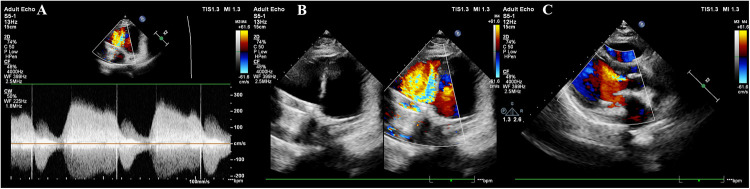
Cardiac ultrasound findings at 32 weeks of gestation. (A) Mechanical mitral valve with anterograde peak velocity (Vmax) of 2.4 m/s and mean pressure gradient (PGmean) of 6 mmHg; Tissue Doppler imaging shows mitral annular diastolic motion (e′ > a′). (B) Mechanical tricuspid valve with stable frame but restricted motion of one leaflet, demonstrating accelerated anterograde flow (Vmax = 2.7 m/s, PGmean = 17 mmHg). No significant perivalvular abnormalities are observed. The aortic valve appears thickened with hyperechoic echoes and incomplete closure. (C) Thickened aortic valve with hyperechoic echoes, incomplete closure, and mild regurgitation.
